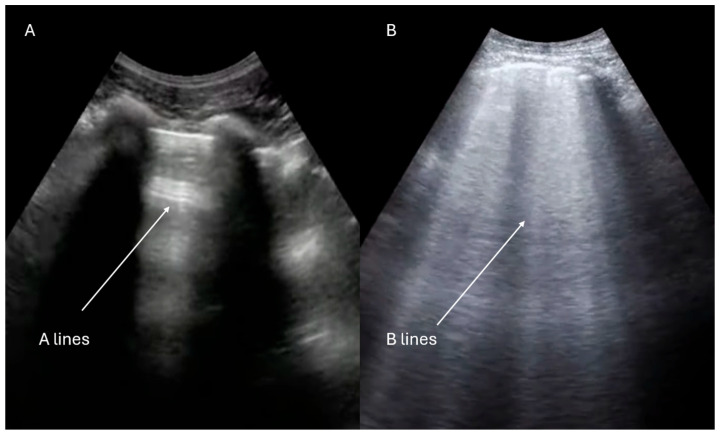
Lung ultrasound in B-mode. (A) A-pattern, characterized by horizontal A-lines. (B) B-pattern, characterized by vertical B-lines.
